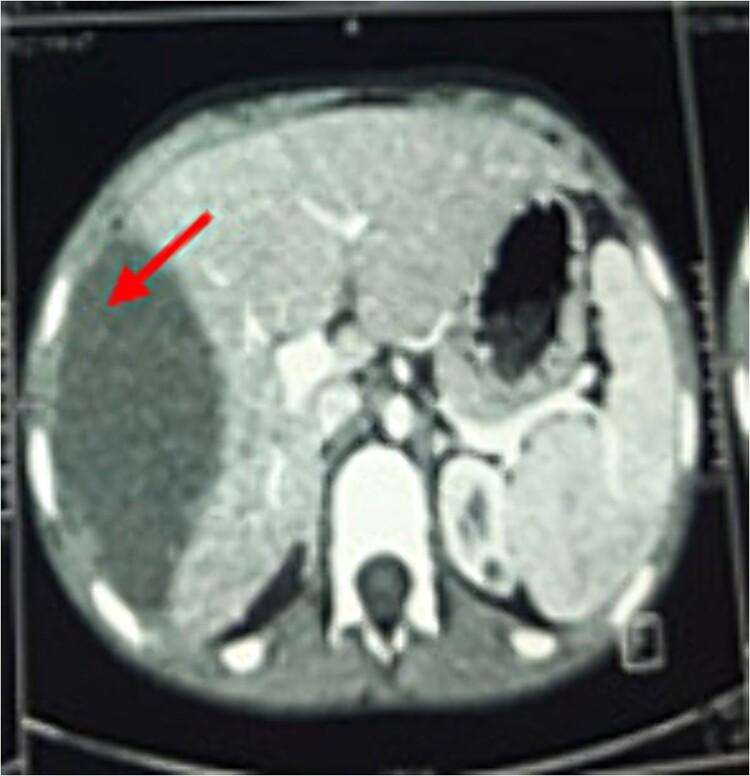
Axial chest CT view of the same hydatid cyst.
